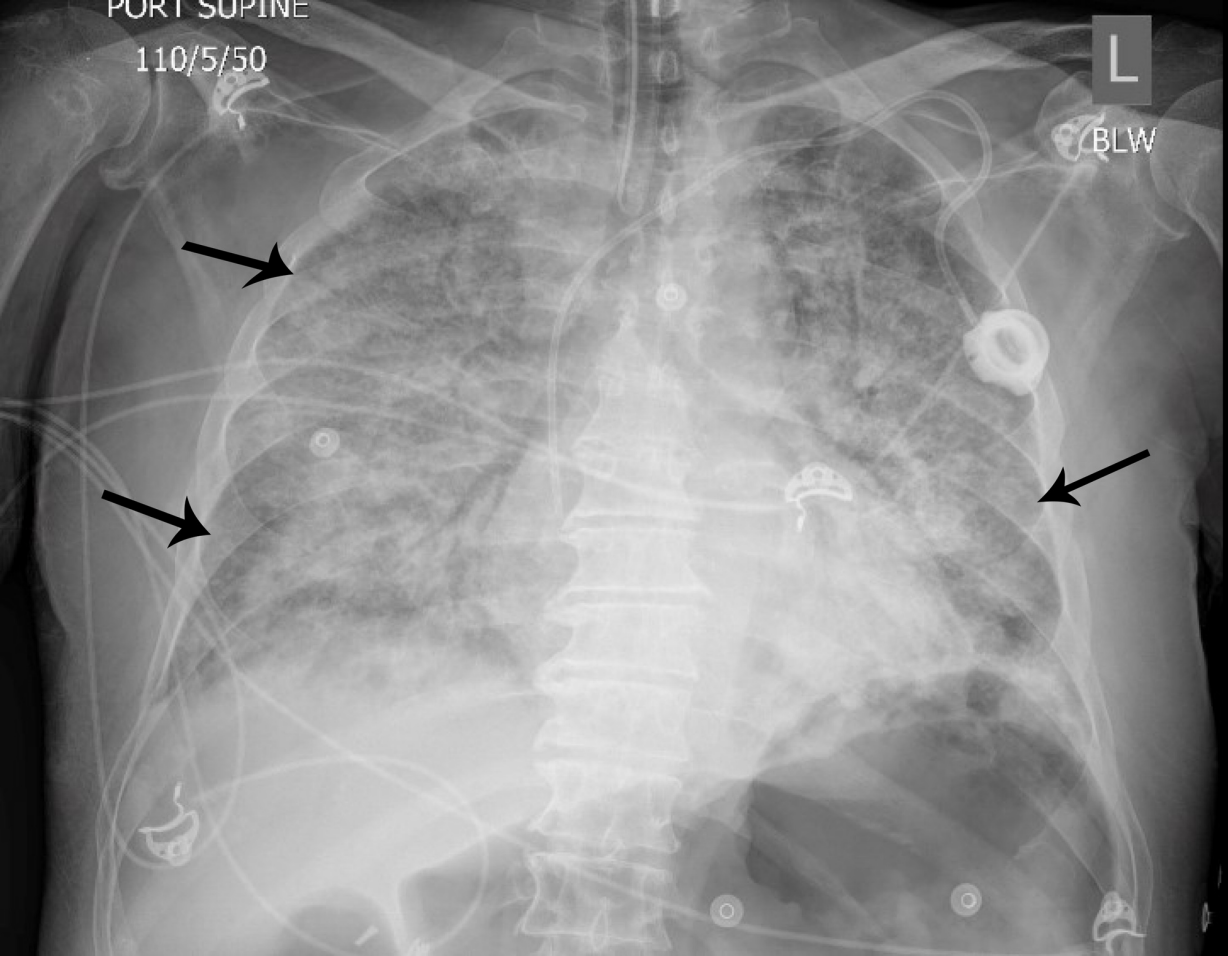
CXR shows diffuse bilateral lung infiltrates (black arrows) CXR, chest X-ray
